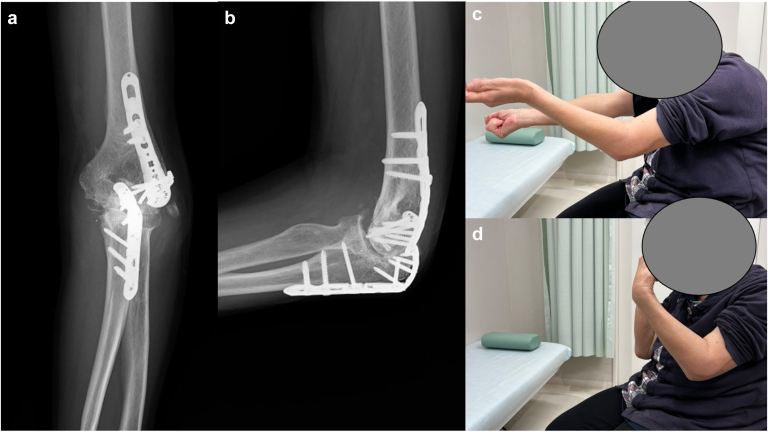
Plain radiographs and clinical photographs after resection of heterotopic ossification. (a) Anteroposterior radiograph, (b) lateral radiograph, (c) extension of the elbow showing −50°, (d) flexion of the elbow showing 85°.
